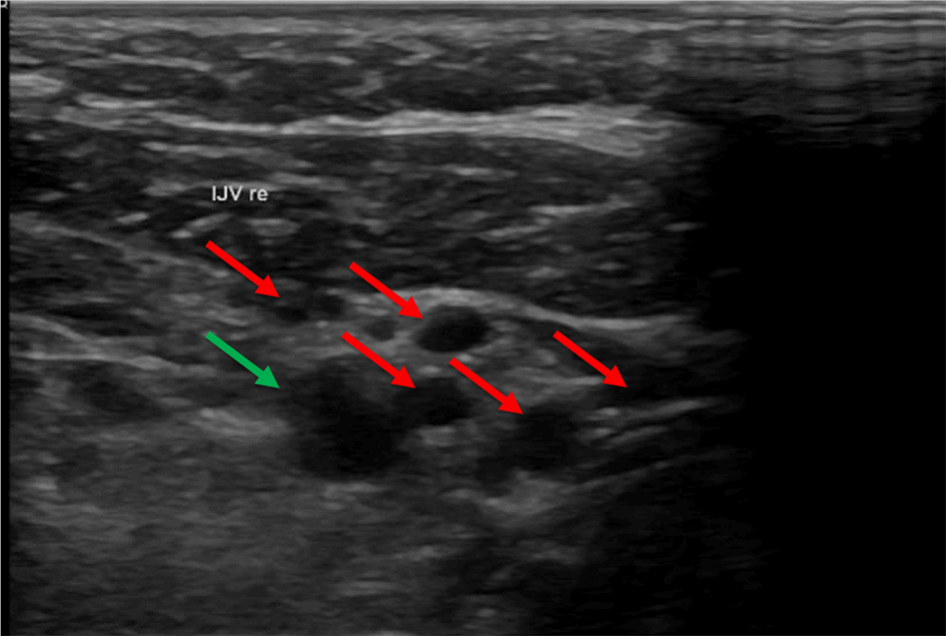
In this ultrasound image the carotid artery (green arrow) is surrounded by many collateral veins (red arrows) due to occlusion of the IVJ. In this ultrasound, the carotid artery is in the upper right part of the image. The small vessels surrounding the artery are collateral veins. The internal jugular vein (IJV) was absent.
